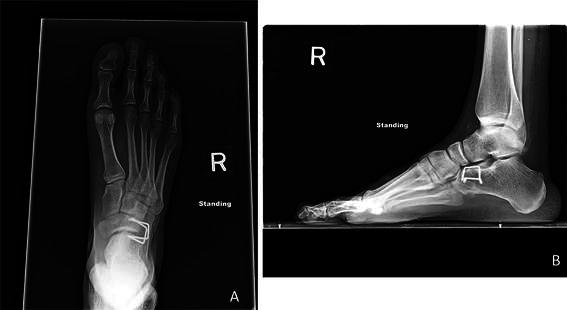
Weightbearing radiographs of right clubfoot in same patient as in Fig. 1: a anteroposterior view and b lateral view
